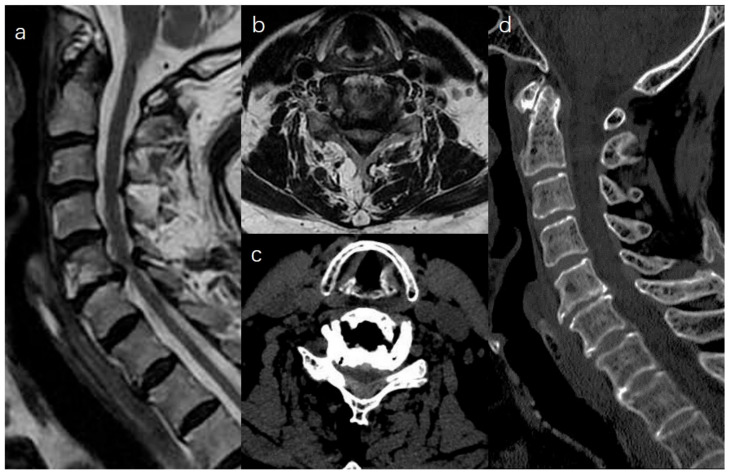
Preoperative MRI showing central canal stenosis at C5–C6 (a,b). The spinal cord was compressed by a herniated disc and hypertrophied LF on CT scan (c,d).
